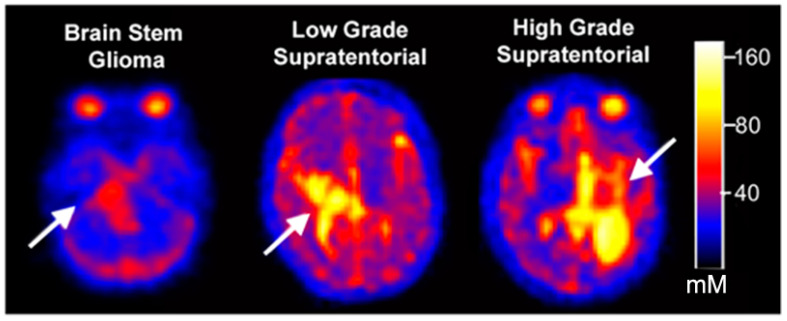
Sodium MRI images demonstrating relative total sodium concentration (TSC) between three different types of pediatric gliomas. There is reduced relative TSC in the pediatric diffuse intrinsic pontine glioma (DIPG) compared to the supratentorial low-grade and high-grade gliomas. There is no difference in relatively high TSC between the low-grade and high-grade gliomas (arrows point to the tumors).
